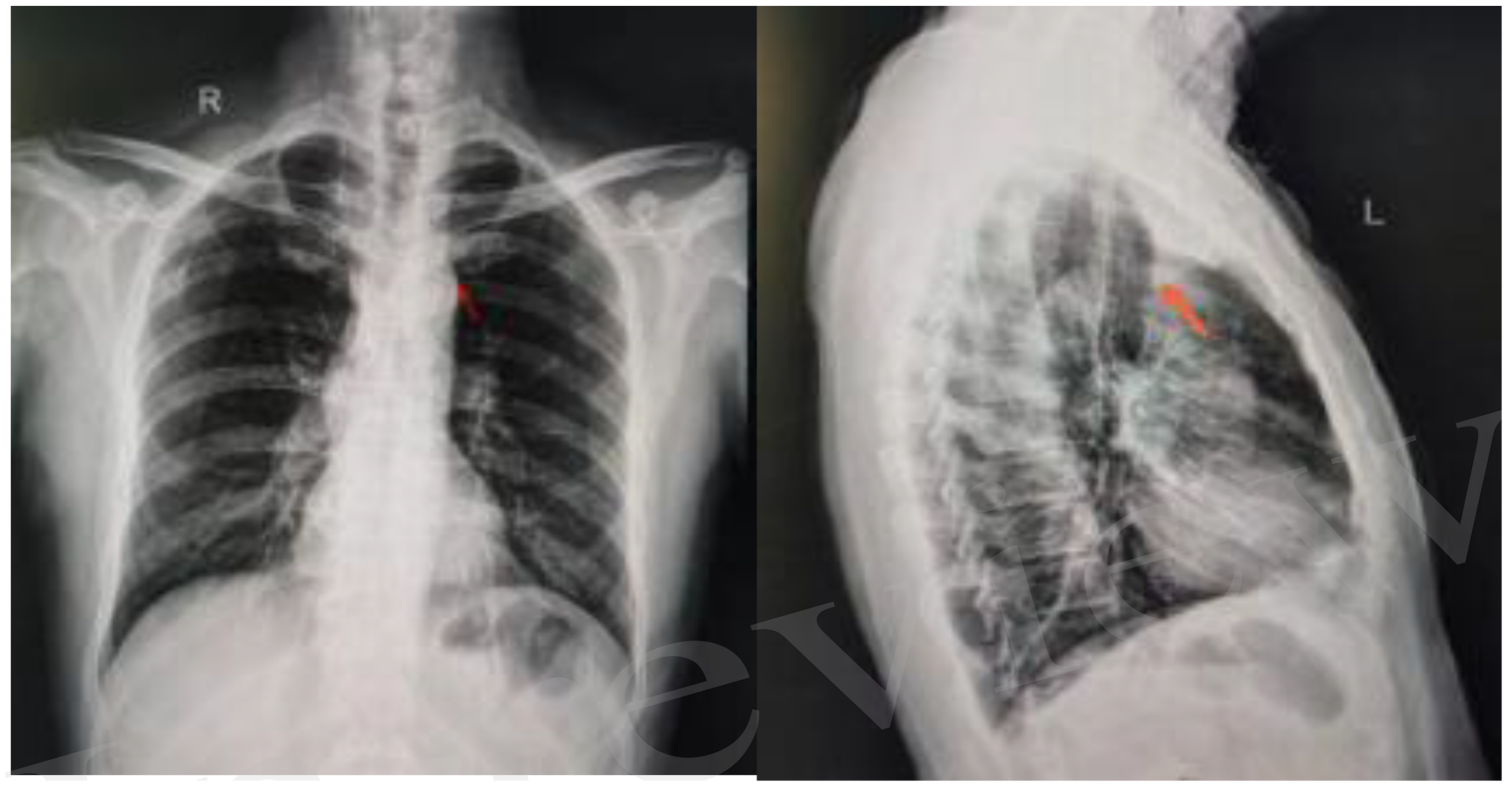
Chest x-ray in the frontal position of the chest with the catheter end under the 1st anterior rib on the left side lateral chest view (right) catheter ends at the lower edge of the 1st anterior rib on the left side.
